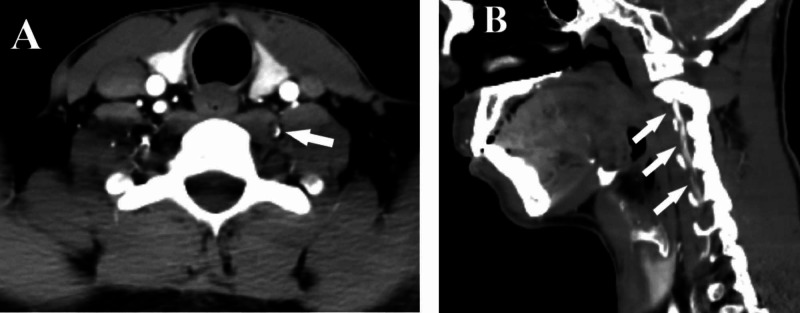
CTA findings – case 1 CTA of cervical arteries showing left vertebral artery intramural hematoma looking crescent-shaped (arrow) in the axial view (A), while it looks spiral (arrows) along (V1-V3) segments in the sagittal reconstructed view (B) CTA: computed tomography angiography
